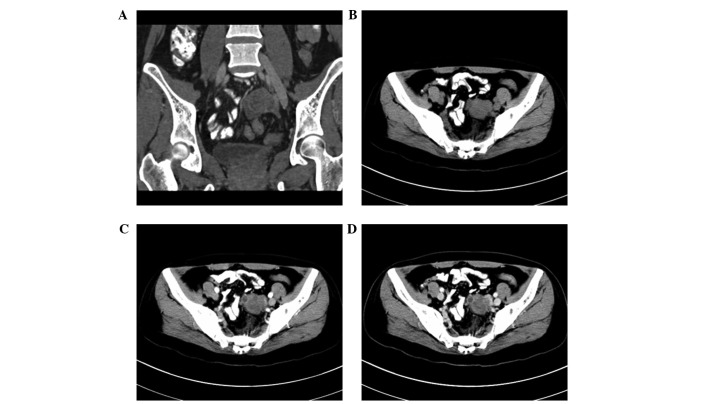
Contrast-enhanced CT image of the pelvic masses in the present case. (A) Coronal image of the pelvic masses. (B–D) A mixed density pelvic lobulated mass was observed from. Moderately non-homegeneous enhancement was observed in arterial phases. CT, computed tomography.
